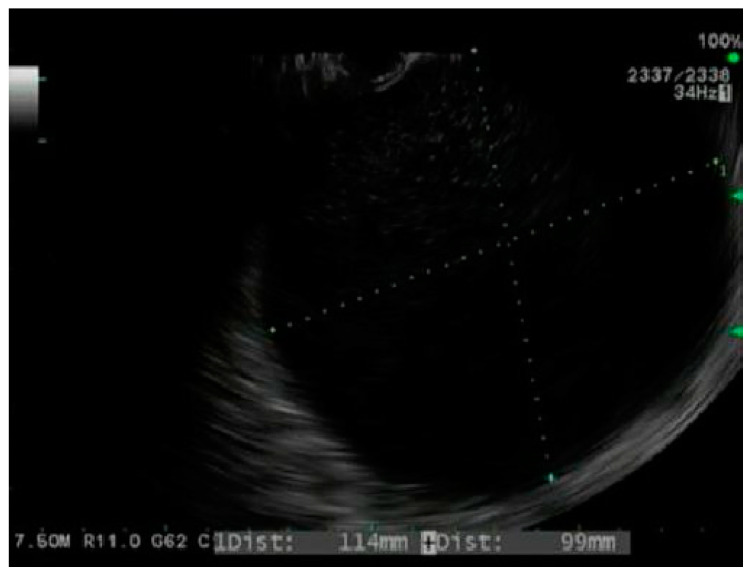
Endoscopic ultrasound image of a pseudocyst.
